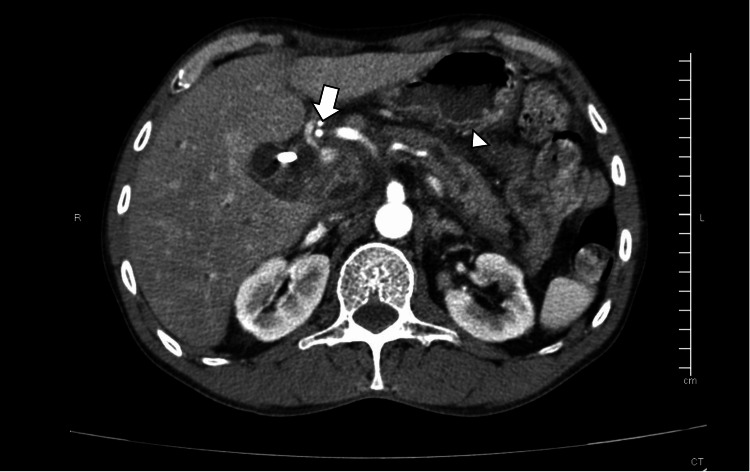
Axial contrast-enhanced CT scan showing a biliary stent (arrowhead) within the common bile duct and peripancreatic fat stranding with edema (arrow), consistent with acute pancreatitis CT: computed tomography
